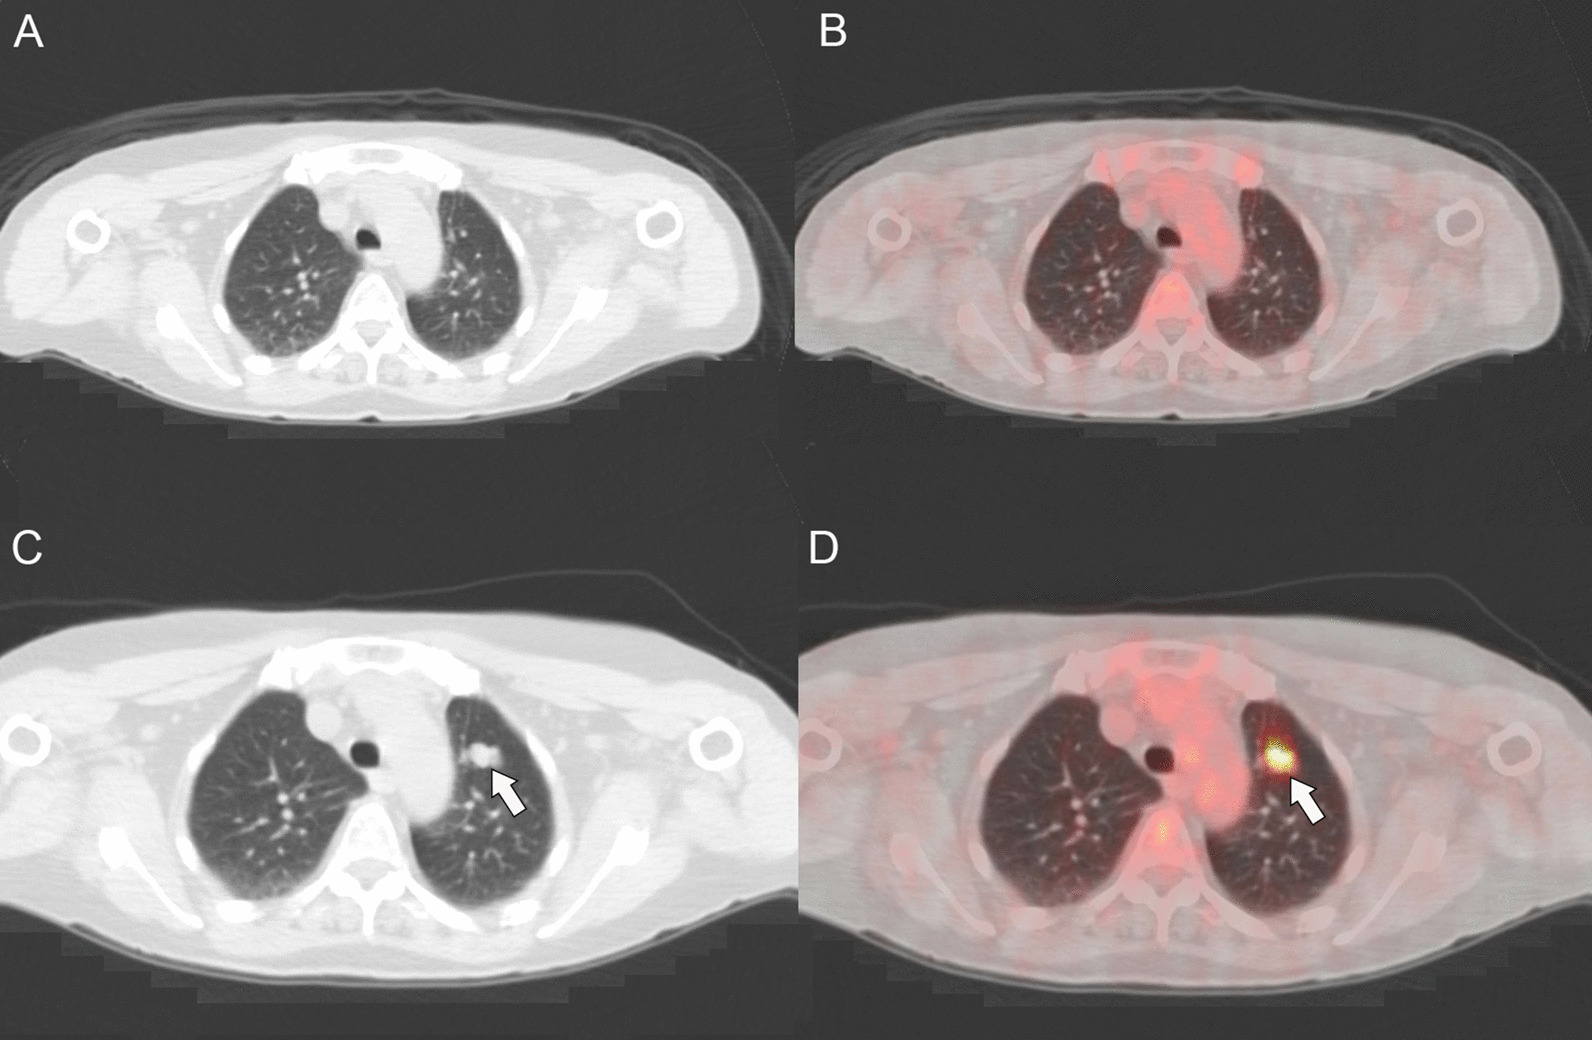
The CT (a) and PET/CT (b) performed November 2013 do not demonstrate any metabolically active metastases or pulmonary nodules. The subsequent CT (c) and PET/CT (d) demonstrate a metabolically active pulmonary nodule in the anterior segment of the left upper lobe (arrows), consistent with a pulmonary metastasis
